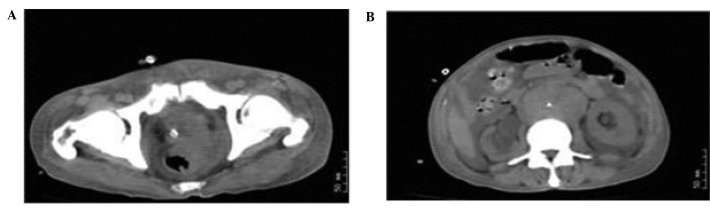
Computed tomography of the abdomen and pelvis. (A) The tumor infiltrated outside of the rectum. (B) The presence of hydronephrosis, retroperitoneal lymph node metastasis and seroperitoneum in the patient.
